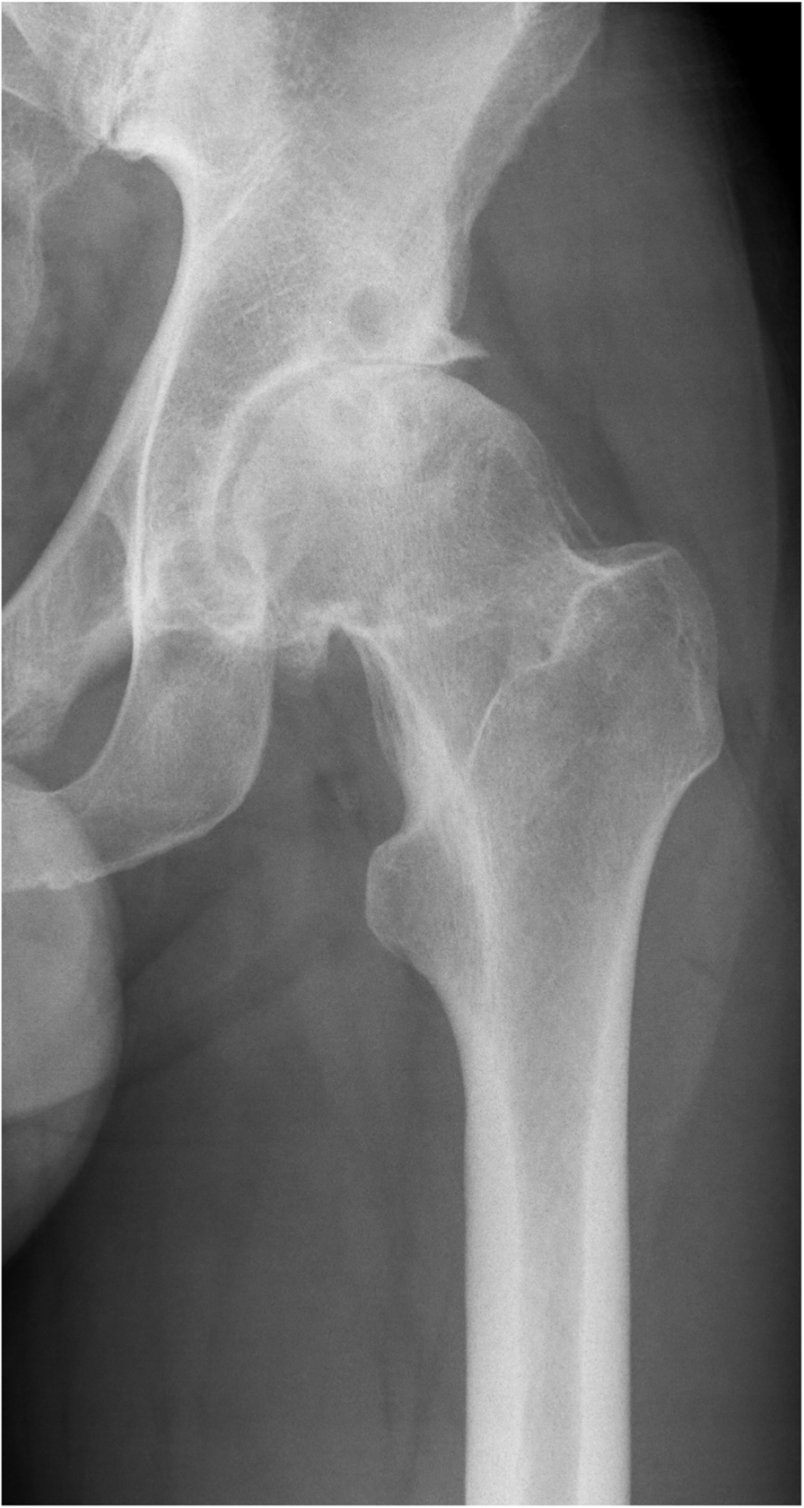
Preoperative x-ray of severe osteoarthritis
